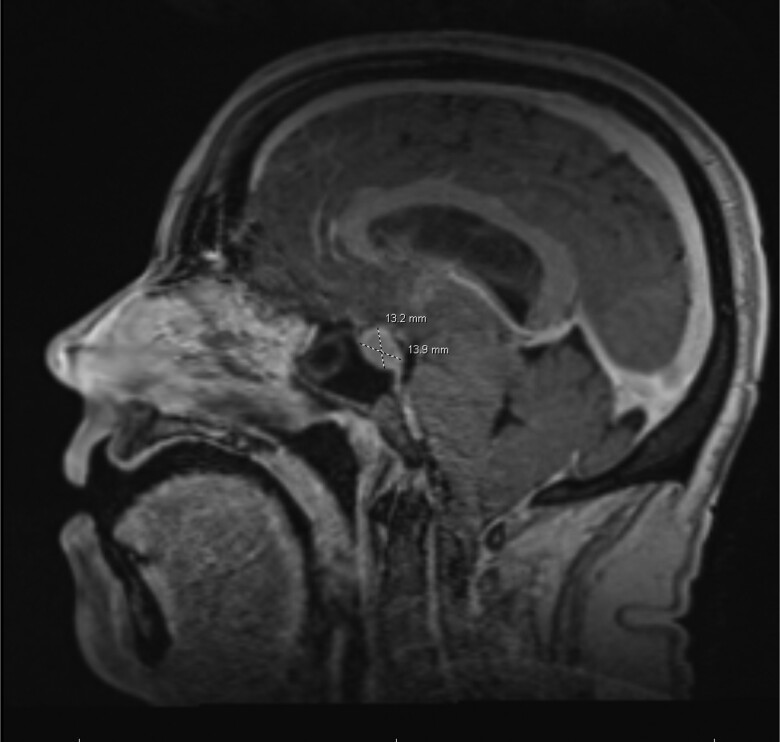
Magnetic resonance imaging brain with without contrast; T1 sagittal image showing the pituitary tumor 13.9 mm anteroposterior.
